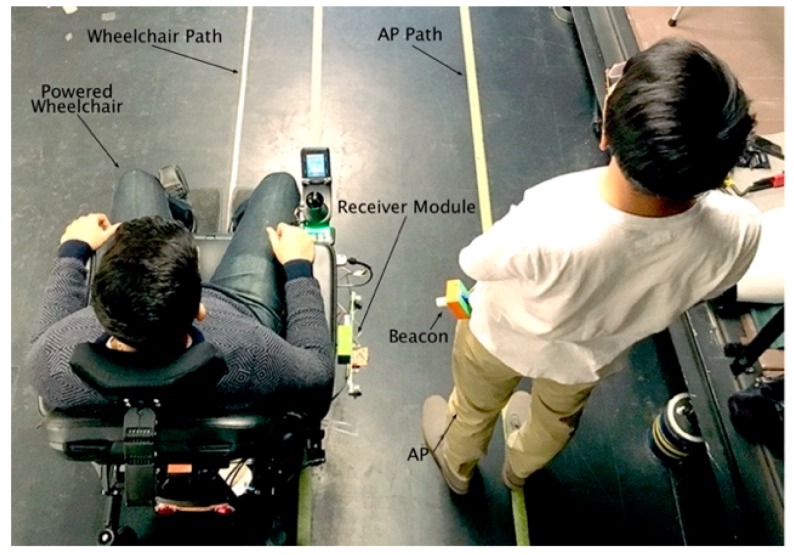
Ultrasonic tethering system performance test setup.
